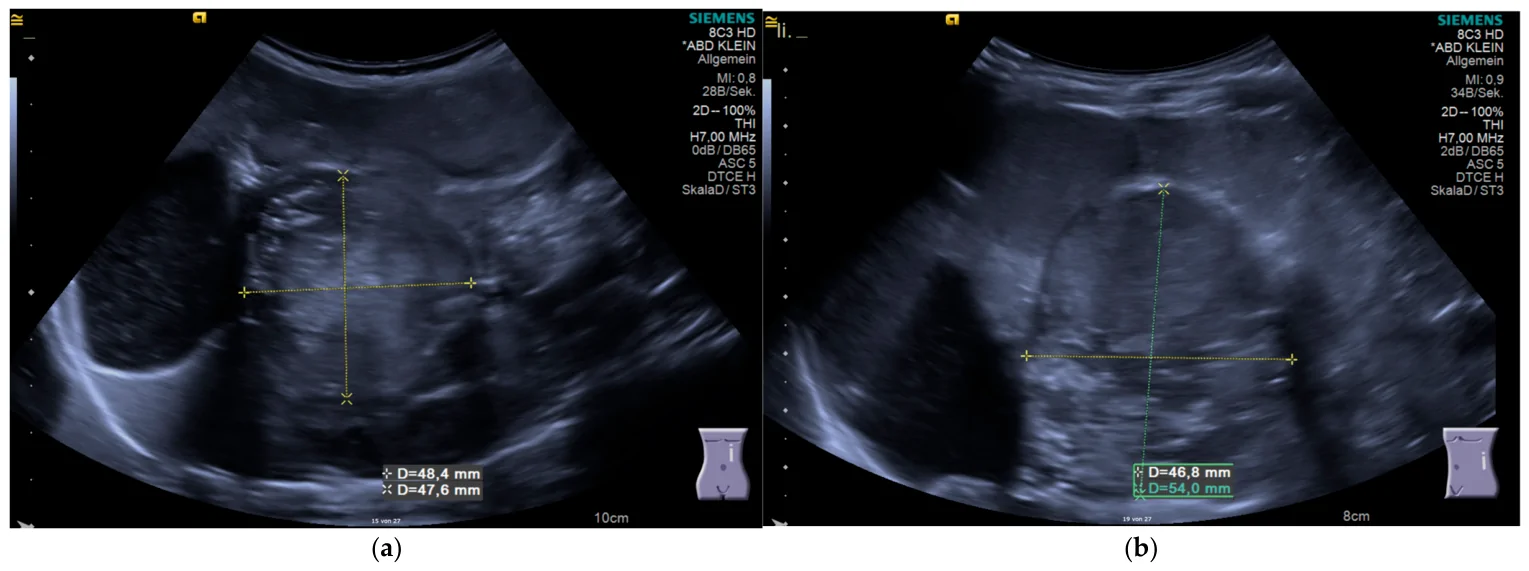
(a,b) Renal ultrasound. (a,b) Visualization of a mass measuring 48 × 47 × 54 mm in the left adrenal gland in two different longitudinal sections of the retroperitoneal space. Imaging was performed using an ACUSON S2000 ultrasound system (Siemens Healthineers, Erlangen, Germany). (University Clinic and Polyclinic for Diagnostic Radiology, Pediatric Radiology, University Hospital Halle; Dr. C. Kunze).
